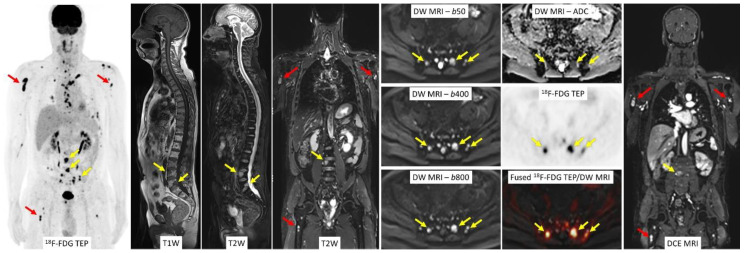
Hybrid PET/MRI provides simultaneous acquisition of morphological, functional, and metabolic information. Focal lesions localized in both the axial skeleton (yellow arrows) and the proximal appendicular skeleton (red arrows) are clearly depicted on the different images. ^18^F-FDG PET, DW MR, and DCE MR images provide combined information on the activity of the multifocal disease.
